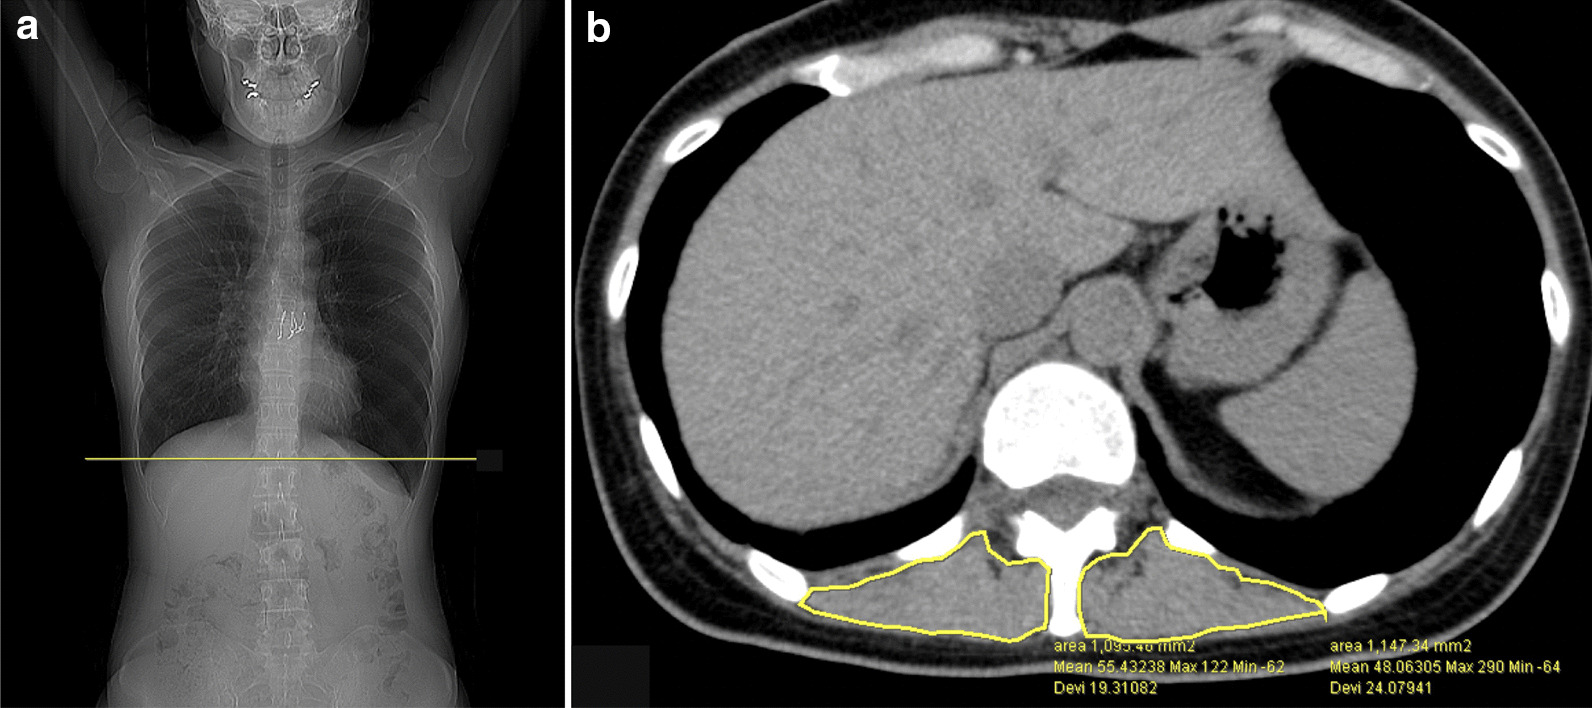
The measurement of the cross-sectional area (CSA) of erector spinae muscle (ESM) in thoracic CT. (a) The image in thoracic CT at the lower margin of the 12th thoracic vertebra was viewed with WeVIEW Z-edition (Hitachi, Ltd, Tokyo, Japan). (b) The border of ESM-CSA was outlined (shown in yellow lines), and the area was automatically calculated
